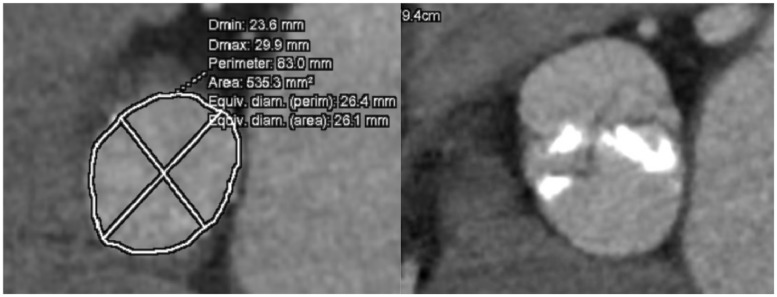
Computed tomography scan reconstruction of the aortic valve annulus of the bicuspid aortic valve.
